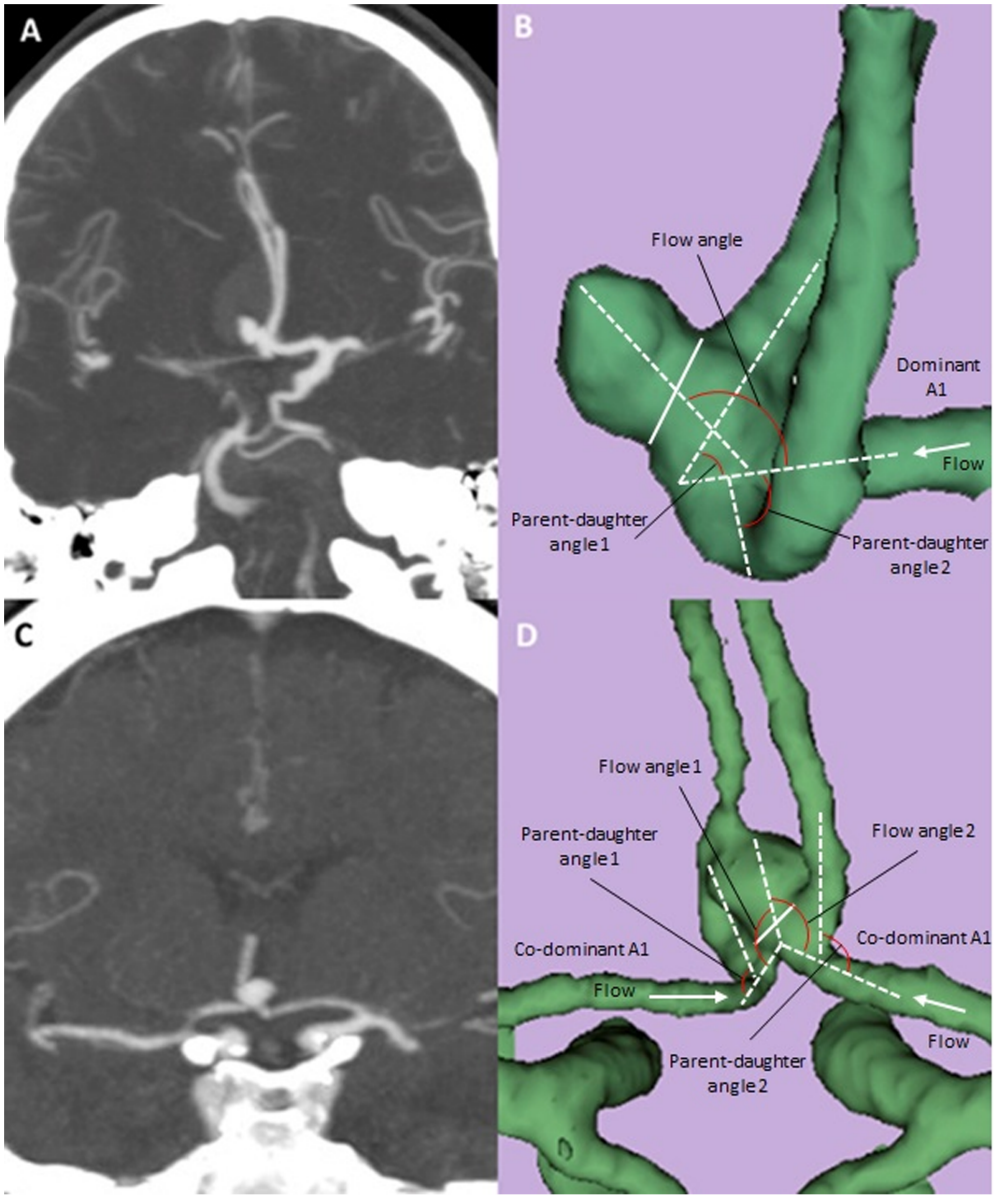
Computed Tomography Angiogram (CTA) and three-dimensional models of anterior communicating aneurysms with a dominant A1 or with co-dominant A1’s. Coronal MIP images are shown for an ruptured AcoA aneurysm with a dominant left A1 (A) and an unruptured AcoA aneurysm with co-dominant A1’s (C). The corresponding 3-D reconstructed images from Slicer are shown in B and D with definitions of morphological parameters. Solid line (B and D) indicates aneurysm neck.
